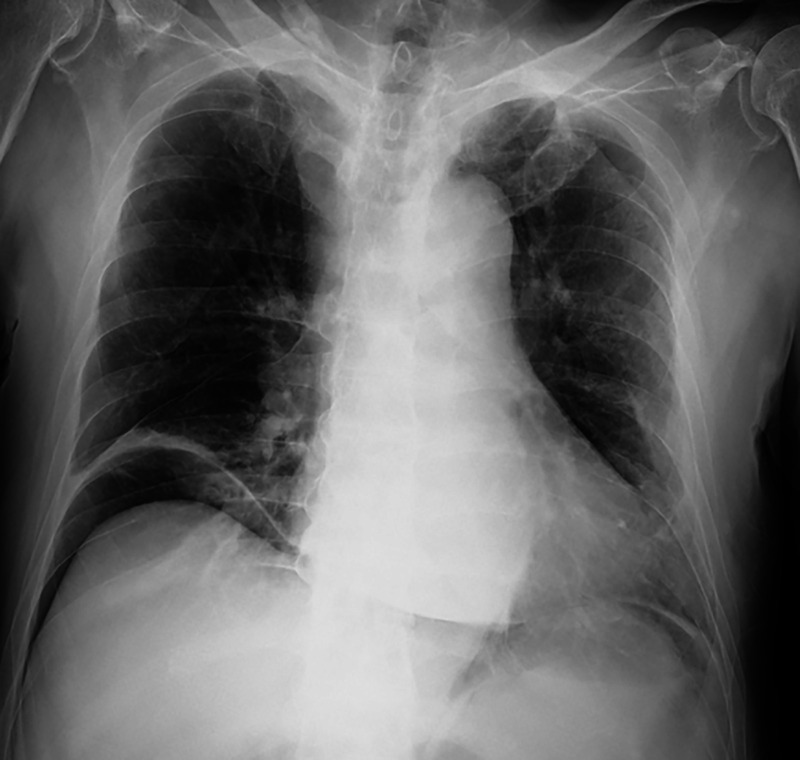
Chest radiograph shows free gas in the bilateral subdiaphragmatic region.
